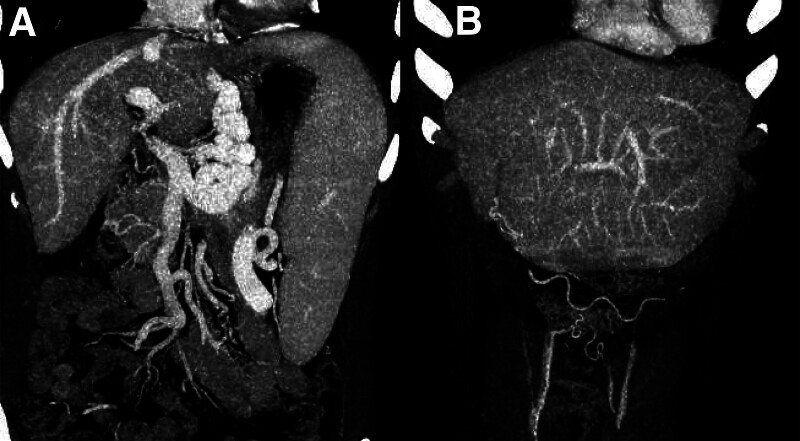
CTV Images. (A) Coronal view showed extensive tortuous dilated veins in the esophagus and gastric varices. (B) Coronal view showed recanalization of the umbilical vein extending to the umbilical region and superficial abdominal varices. CTV = computed tomography portal venography.
